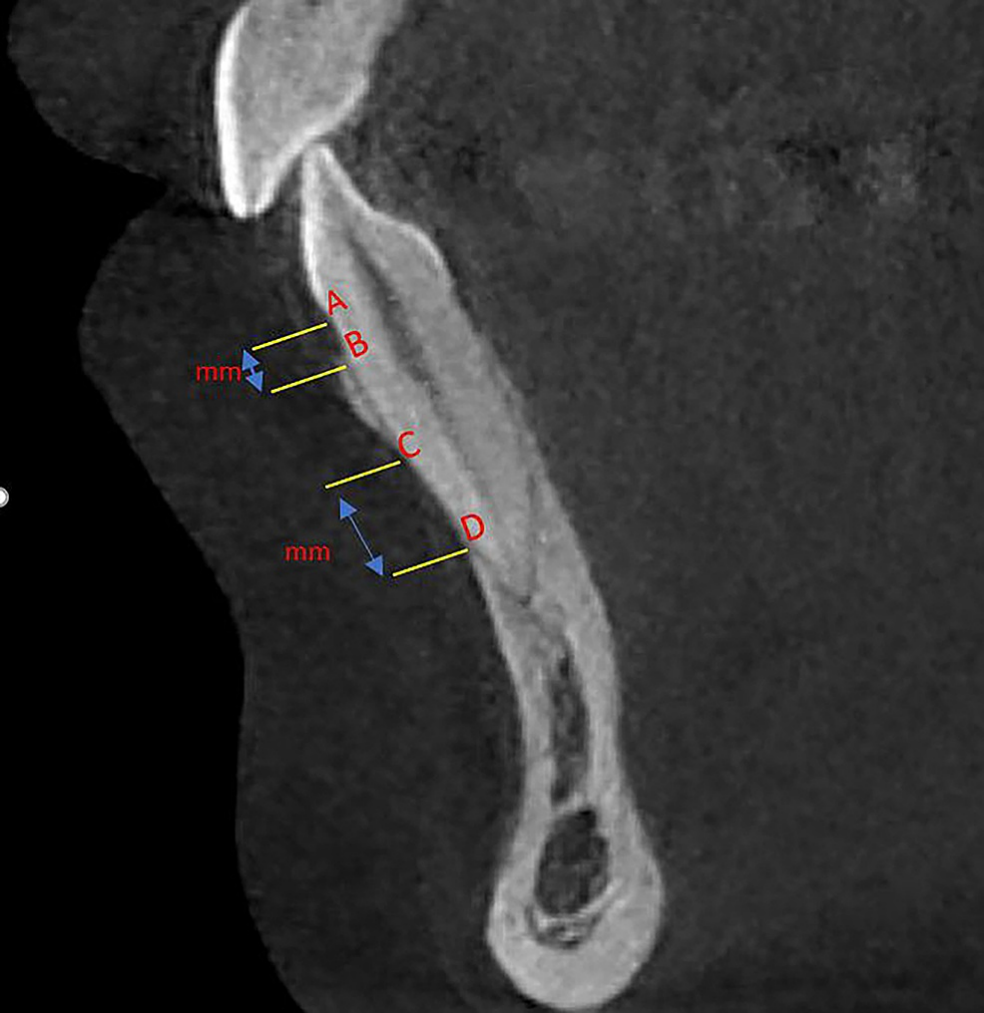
Measuring the dimensions of bone defect based on the sagittal view of the CBCT data. A: Cementoenamel junction (CEJ) at the labial side, B: Alveolar crest at the labial side, C: Coronal border of a fenestration, D: Apical border of a fenestration, DC: Distance between A and B measured by the CBCT method (mm), FC: Distance between C and D measured by the CBCT method (mm)
